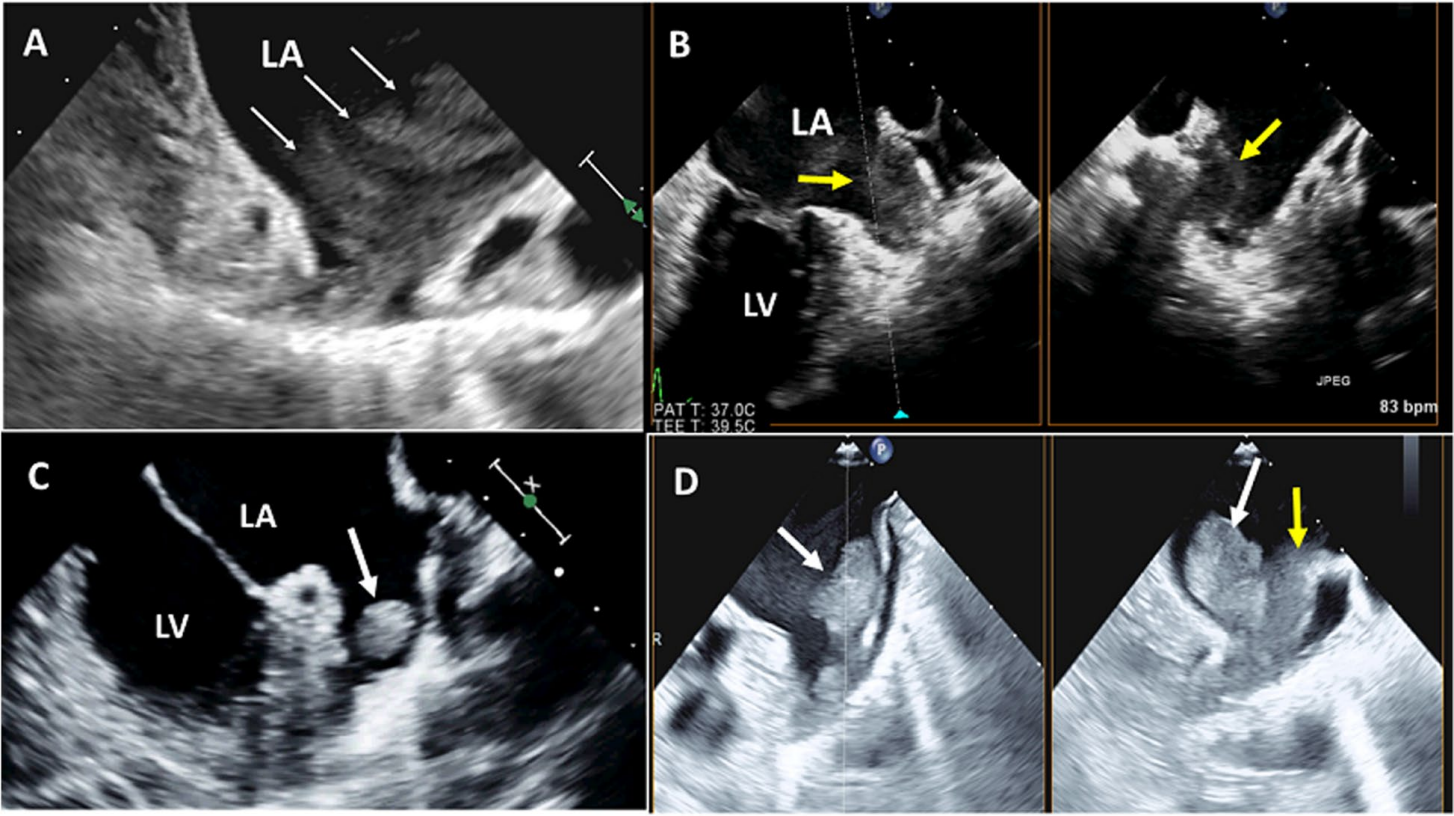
Left atrial appendage (LAA) visualization by two-dimensional transesophageal echocardiography shows the sequel of blood stasis. A Severe spontaneous echo contrast (small white arrows). B X-plane view of LAA shows dense spontaneous echo contrast associated with sludge formation (yellow arrow). C Small rounded LAA thrombus (white arrow). D Large LAA thrombus protruding into the LA (white arrows) associated with sludge (yellow arrow)
